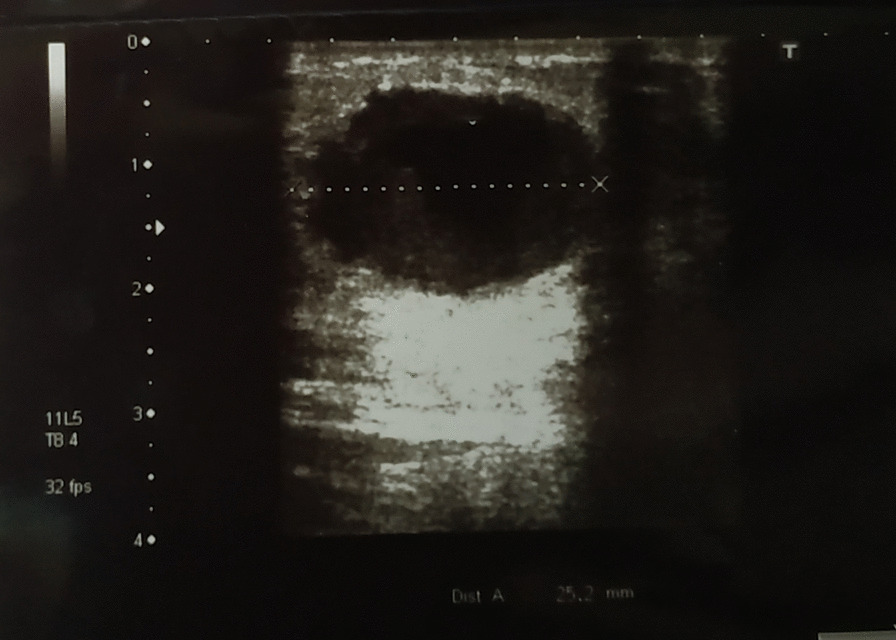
Ultrasonography of the breast demonstrating a well-defined hypoechoic cyst with heterogeneous components
